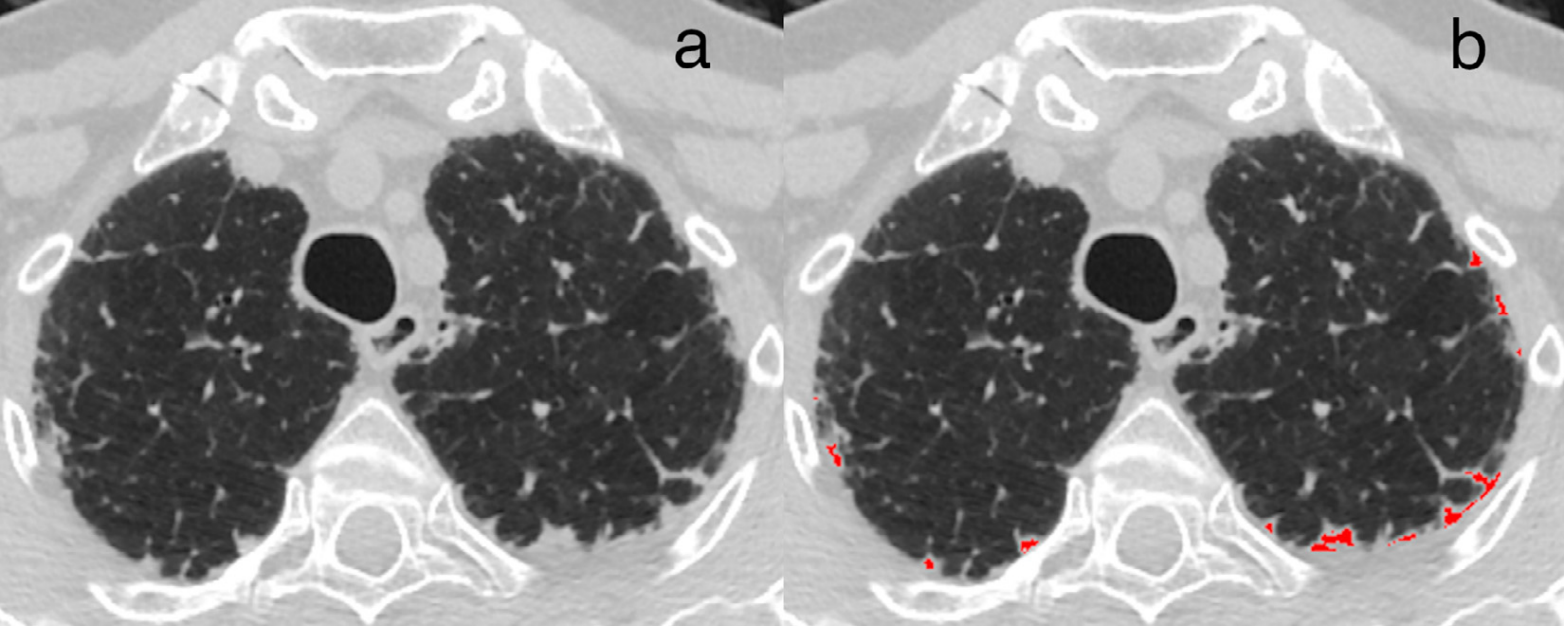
Computerised segmentation of PPFE. (a) Axial CT image demonstrating a patient with marked visual upper-lobe PPFE extent (vPPFE) and computerised PPFE extent (cPPFE) of 8·1%; (b) same axial CT image with the computerised segmentation of PPFE in the lung periphery shown in red. PPFE = pleuroparenchymal fibroelastosis, vPPFE = visual upper-lobe PPFE extent, cPPFE = computerised upper-zone PPFE extent.
